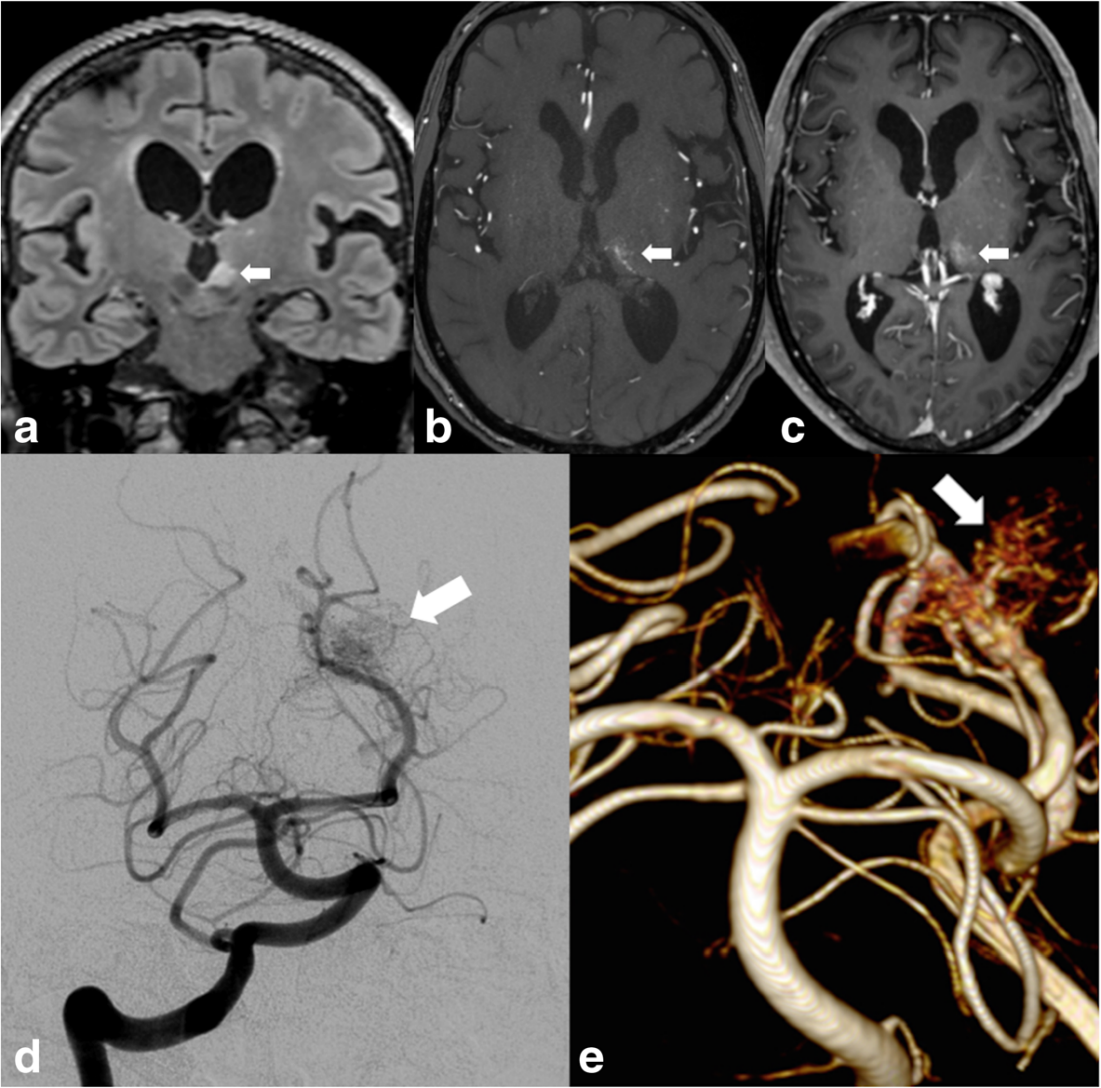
High signal intensity on FLAIR in the left thalamus (arrow in a) associated with a “bag of worms” appearance on 3D TOF (arrow in b). DSA and 3D TOF reformat (e) demonstrate abnormal vessels corresponding to the nidus of an AVM (arrows in c and d)
